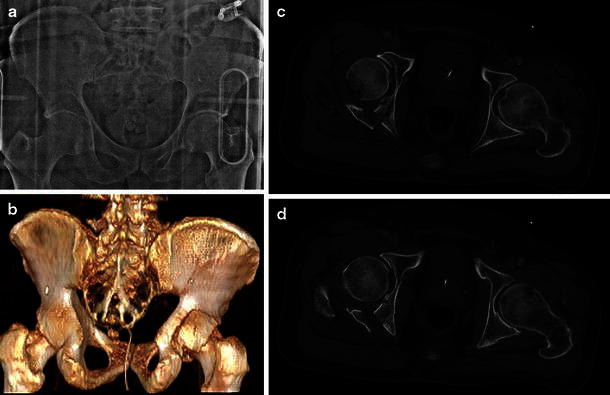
Preoperative a X-ray, b–d 3-D and 2-D CT scans (Male, 73 years, car accident)
